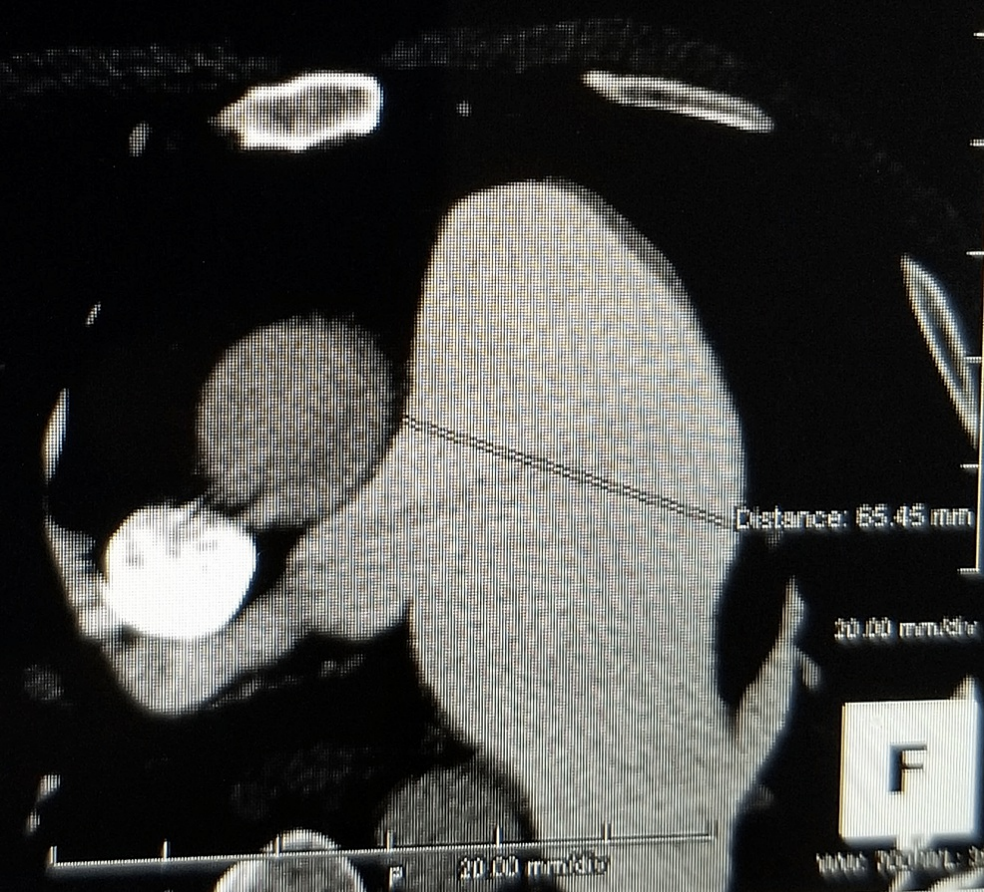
Aneurysmal pulmonary artery as seen on CTA
